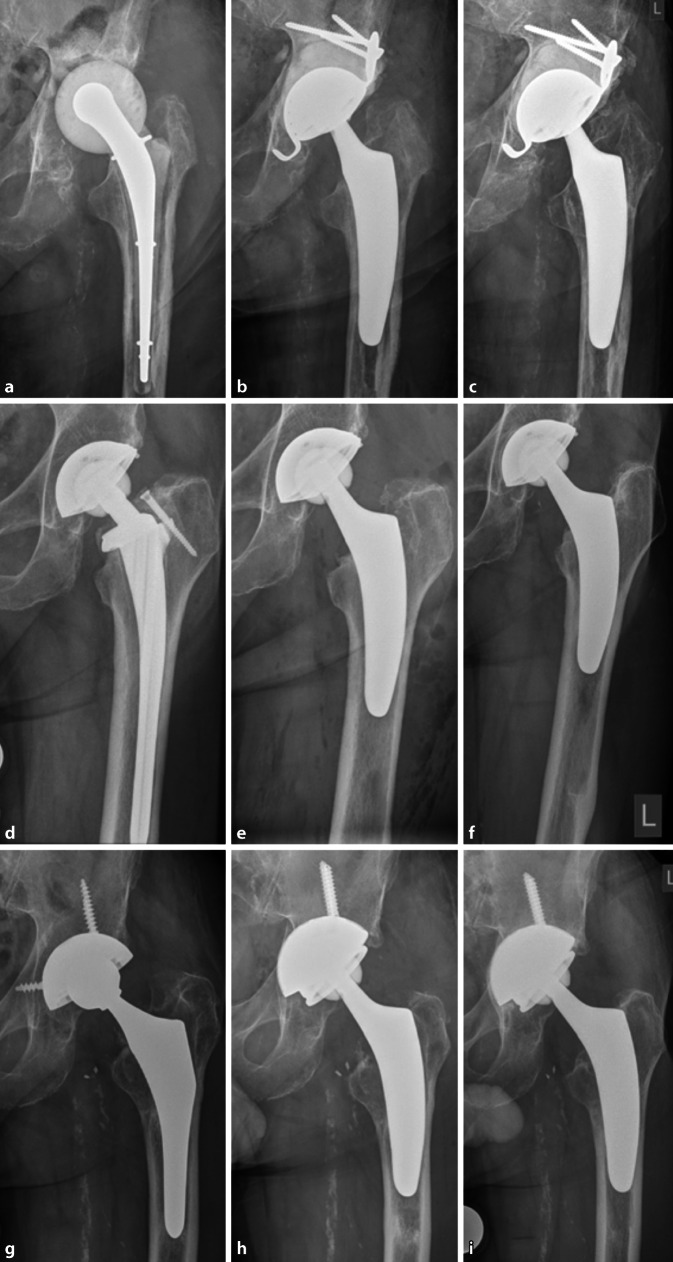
Radiographs of patients 4 (a–c), 5 (d–f) and 6 (g–i), a,d,g preoperatively, b,e,h postoperatively, c,f,i at last follow-up
